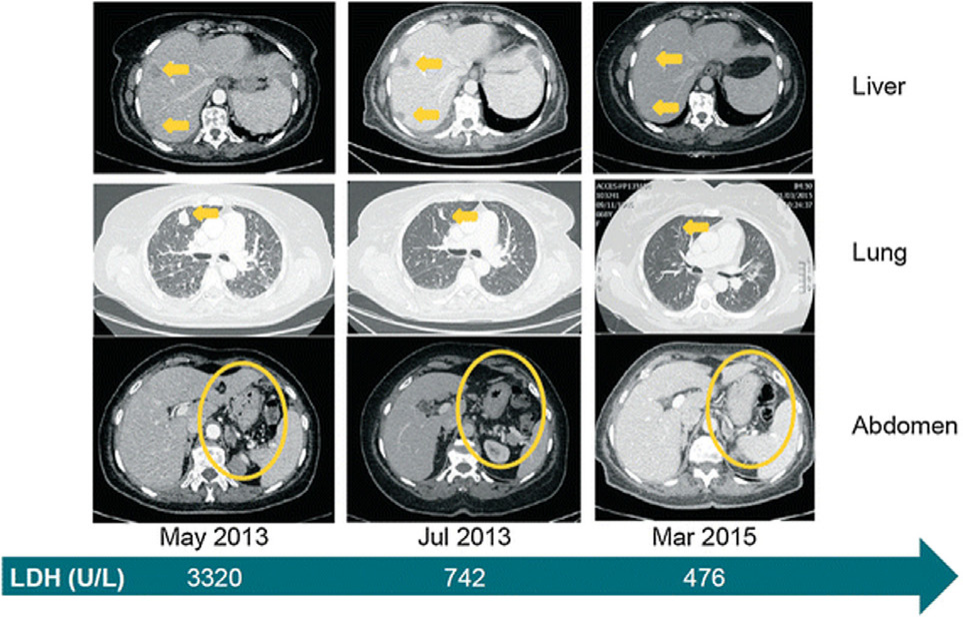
Clinical evidence that treatment with the immune checkpoint inhibitor, nivolumab is effective against metastatic mucosal melanoma. Systemic clinical response to nivolumab treatment. Computed tomography images from baseline (May 2013), 2 months into treatment and approximately 2 years later. *Yellow arrows or circles* indicate metastatic foci and their complete disappearance by March 2015. The *green arrow* depicts the level of LDH at the selected time points. *LDH* lactate dehydrogenase. Reprinted from Ascierto et al. (126) with kind permission by Springer Berlin Heidelberg.
